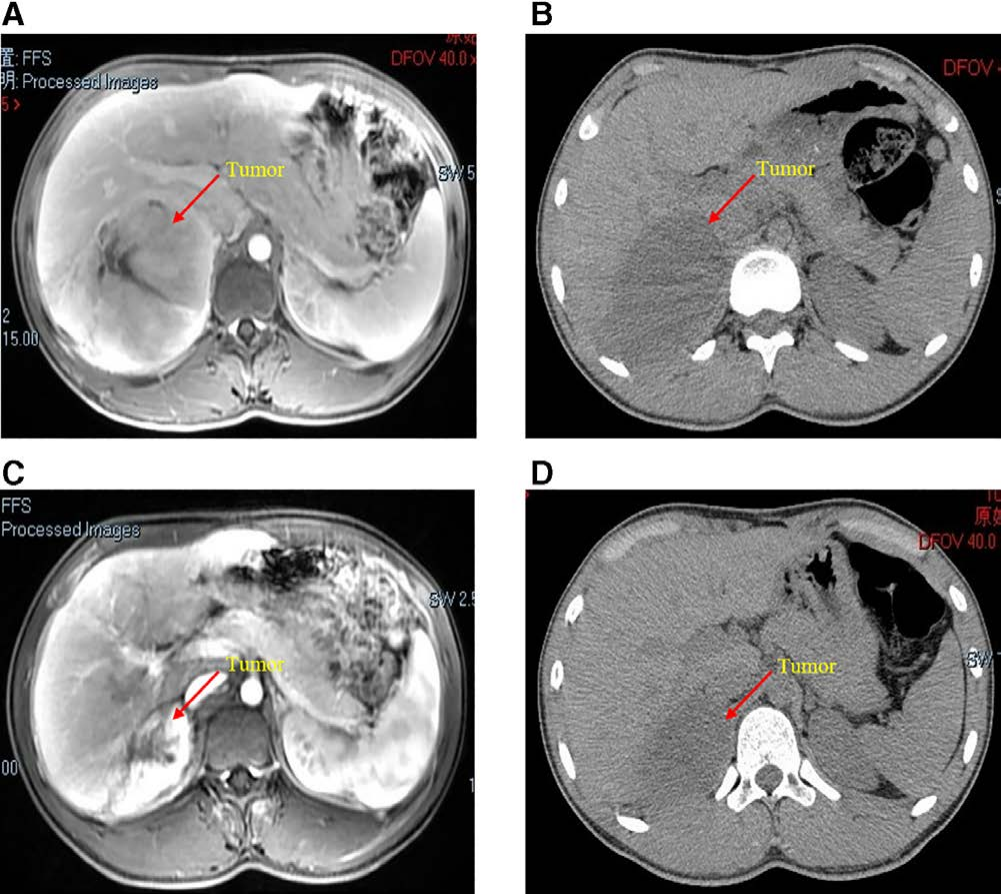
The MRI (A) of the patient shows a recurrent mass in the operative area, which adhered closely to the right lobe of the liver. The CT scans show that the mass gradually shrinks after the second (B), fourth (C), and sixth cycles (D) of neoadjuvant therapy. CT = computed tomography, MRI = magnetic resonance imaging.
